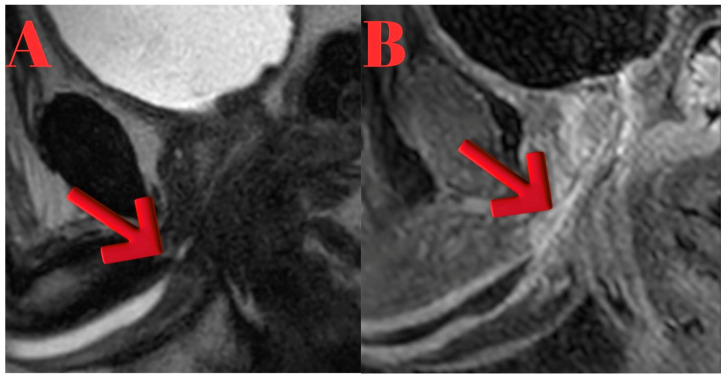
(A): Sagittal T2-weighted MRI showing inflammatory changes and complete obliteration extending from the proximal bulbar urethra to the distal prostatic urethra (red arrow indicating the obliterated segment). (B): Sagittal T1-weighted fat-suppressed post-contrast MRI highlighting fibrosis with contrast enhancement in the strictured segment (red arrow indicating the fibrotic lesion).
